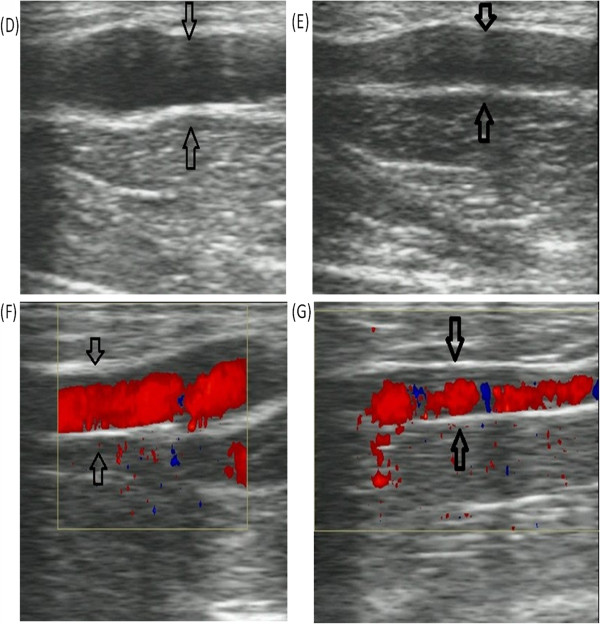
B-type ultrasonography of the cords extending from the axilla to the medial arm. (A) The cords under the axilla present Eco of a tube structure. (B) The cords in the medial arm present the echo of a tube structure. (C, D) Blood is observed in the tube structures of (A) and (B), respectively.
